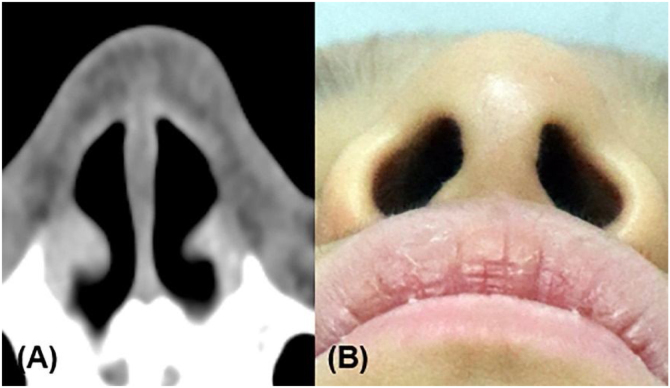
Post-operative 1-year CT scan (A) and clinical photo (B) revealed no evidence of recurrence or residual lesion.
